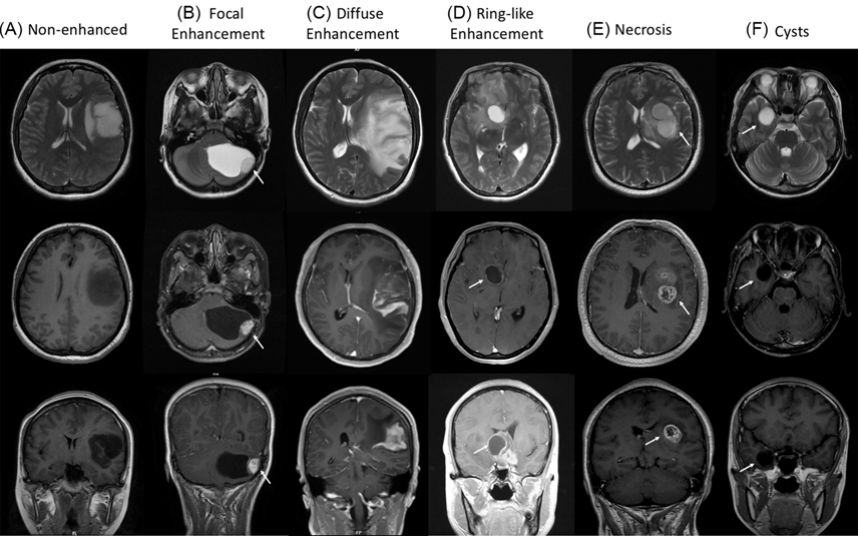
Representative images of classification of contrast enhancement patterns T2-W images (the first line) and contrast-enhanced T1-W images (the second and bottom lines) of different MRI features were exhibited in LGGs. (A) Non-enhanced pattern; (B) Focal enhancement pattern; (C) Diffuse enhancement pattern; (D) Ring-like enhancement pattern; (E) Necrosis; (F) Cysts.
